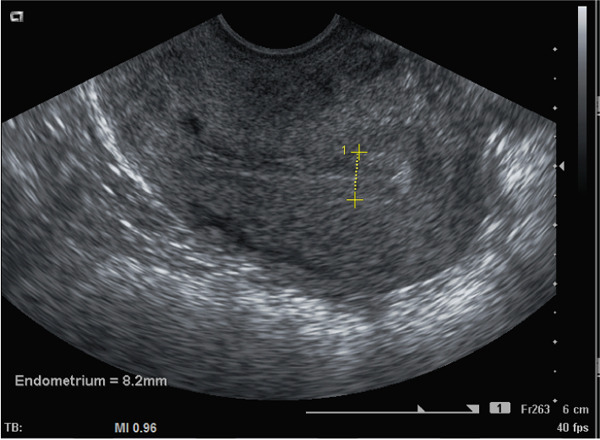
Postoperative transvaginal sonogram image at midcycle after hysteroscopic revision of CSI showing a trilaminar endometrial lining and no endometrial cavity fluid.
